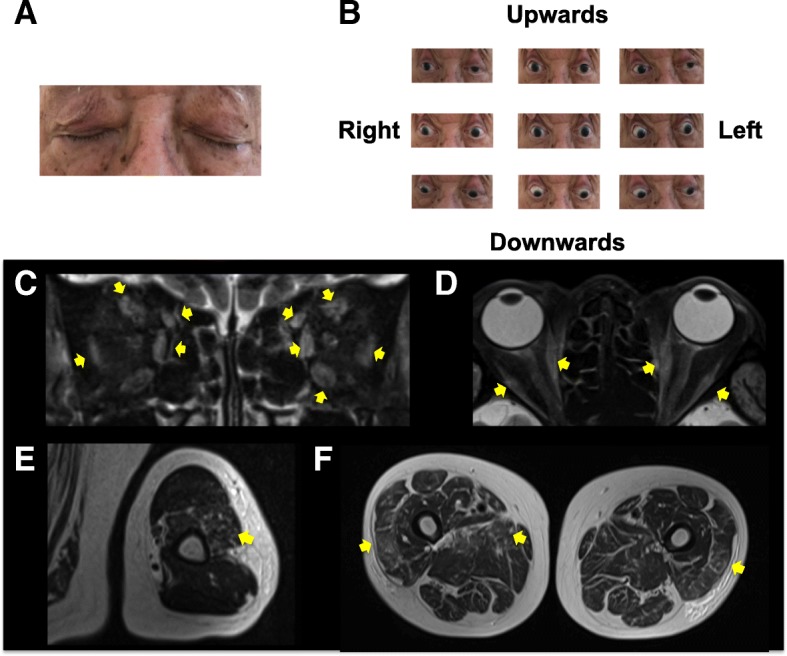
Ptosis, eye movements and muscle MRI in case 1. On admission, case 1 exhibited bilateral ptosis (a), and restricted eye movements, particularly for upward gaze (b). Hyperintense signal and enhancement on short T2 inversion recovery (STIR) in bilateral orbicularis oculi muscle: coronal view (c) and axial view (d), brachial muscle (e) and femoral muscle (f). Yellow arrows indicate inflammation changes in muscles
